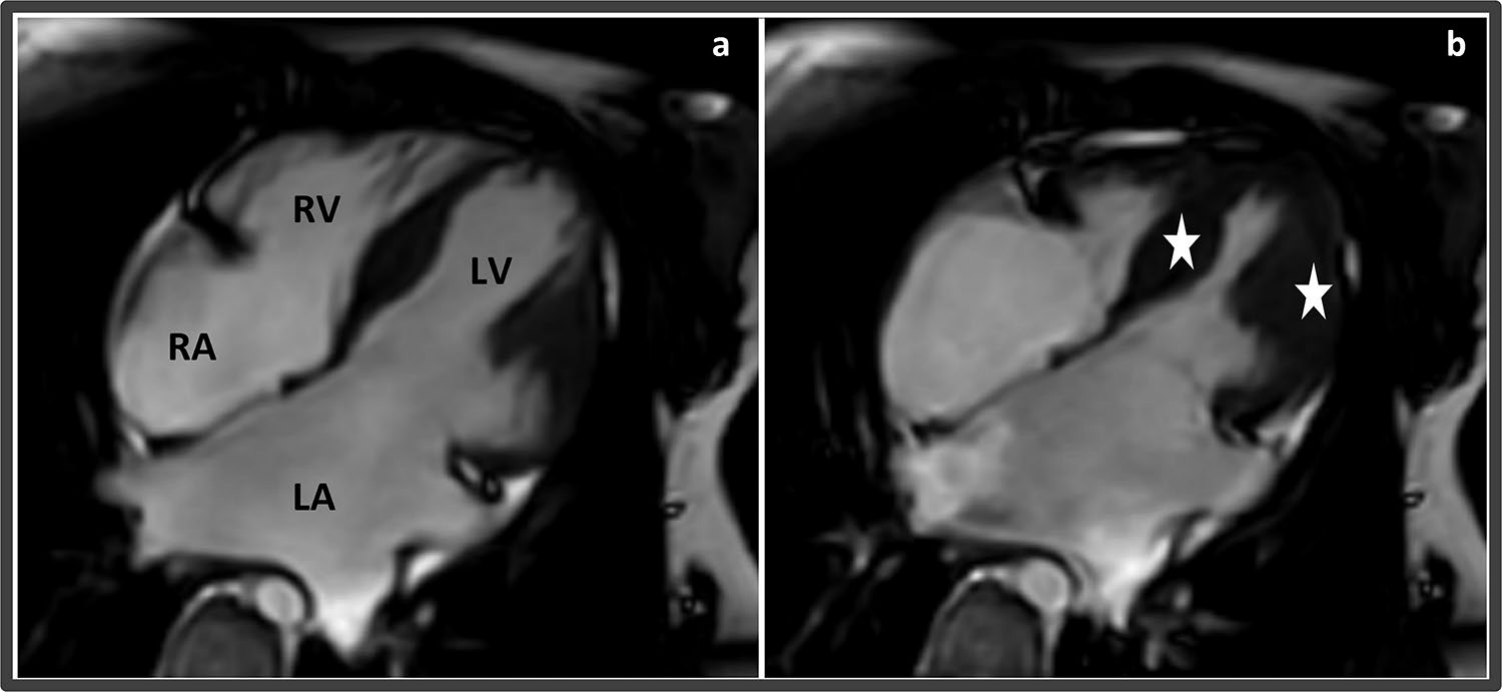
Cardiac magnetic resonance image of proband in F09 with RCM. (a) Cardiac magnetic resonance image of 4-chamber view in diastolic, and (b) systolic phases, showing Bi-atrial dilatation, with preserved systolic function. Mild thickening of the mid-section of interventricular septum and left ventricular free wall is also noted (white stars). *LA* left atrium, *LV* left ventricle, *RA* right atrium, *RV* right ventricle.
